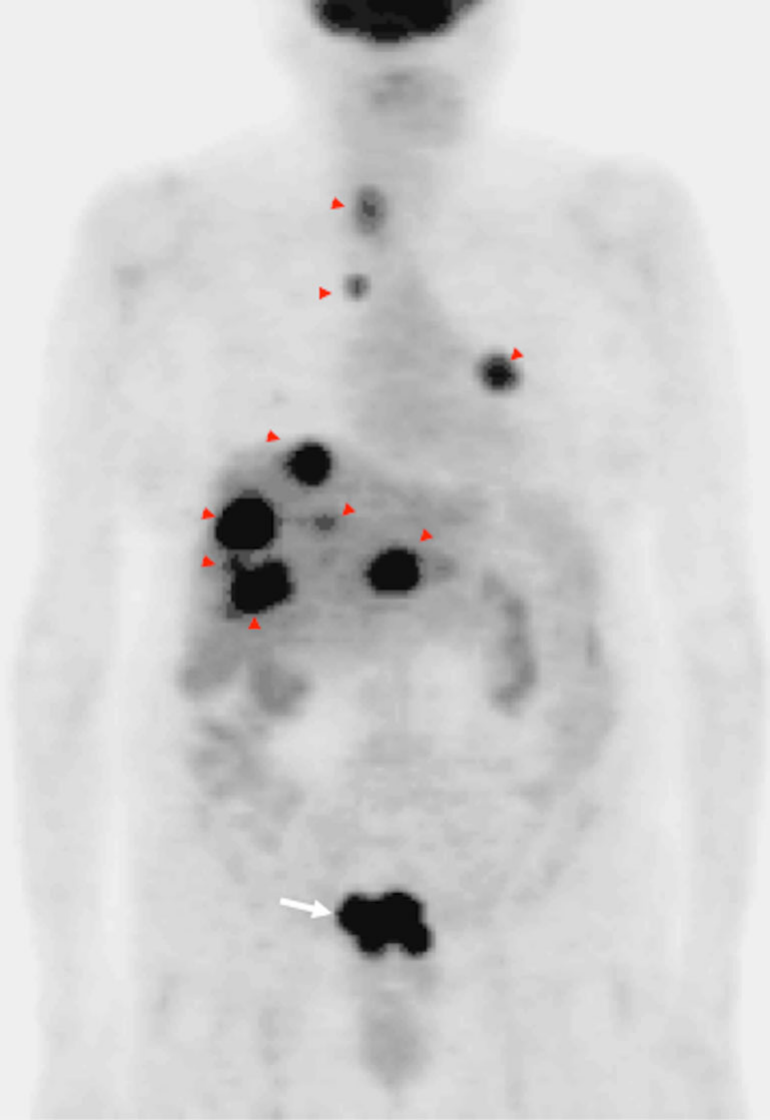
^18^F-FDG-PET in staging rectal cancer. Coronal PET image evidenced a focal area of uptake in the pelvis corresponding to the primary tumour (white arrow). PET also demonstrated multiple liver, lung, and lymph-node metastases (red arrowheads)
